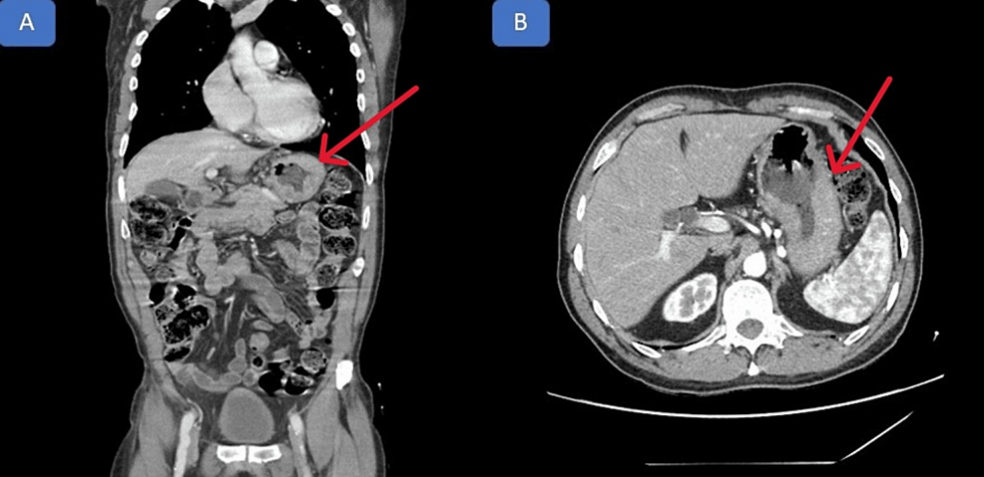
Computed tomography of the abdomen Circumferential parietal thickening of the fundus and gastric body and loss of gastric folds. Coronal plane (A) and axial plane (B).
